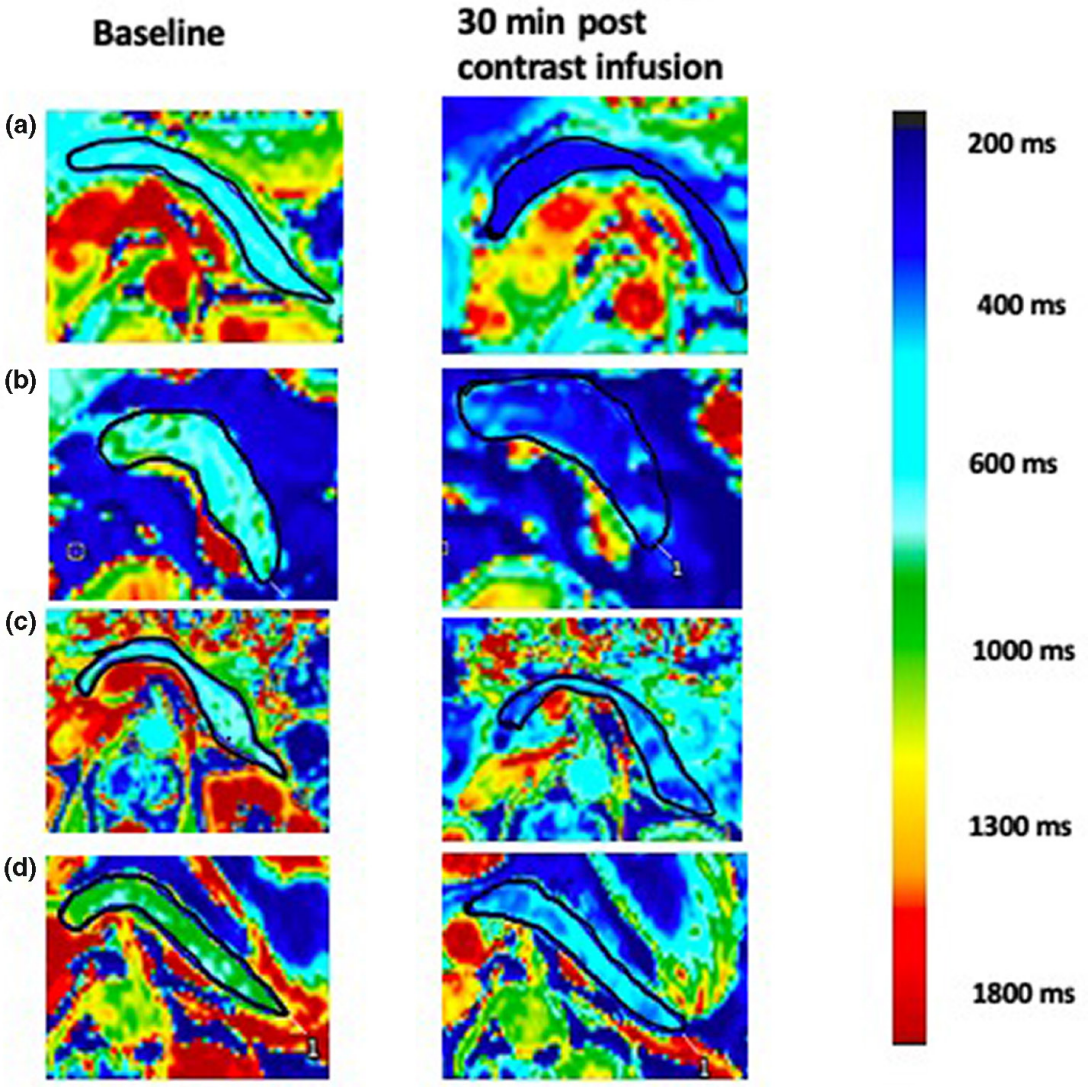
Example pancreatic T1 maps in study participants. Pancreas at baseline (left‐hand column) and 30 min after manganese contrast infusion (right‐hand column) in (a) healthy volunteer (plasma C‐peptide concentration > 500 pmol/L; Ki 35 mL/100 g of tissue/min), (b) individual with type 1 diabetes mellitus and low C‐peptide concentration (362 pmol/L; Ki 27 mL/100 g of tissue/min), (c) individual with type 1 diabetes mellitus and very low C‐peptide concentration (0 pmol/L; Ki 5 mL/100 g of tissue/min) and (d) individual with type 1 diabetes mellitus and very low C‐peptide concentration (32 mol/L; Ki 15 mL/100 g of tissue/min). Colours are assigned to different T1 values on a pixel‐by‐pixel basis. The colour scale shows T1 values corresponding to the different colours. T1 values represent the time constant for longitudinal relaxation time which is measured in milliseconds and is a standard measure for magnetic resonance imaging. Note the differences in the appearance of pancreas in participants with different C‐peptide concentrations. The areas with reduced enhancement post contrast represent reduced manganese uptake and likely areas of beta‐cell loss.
